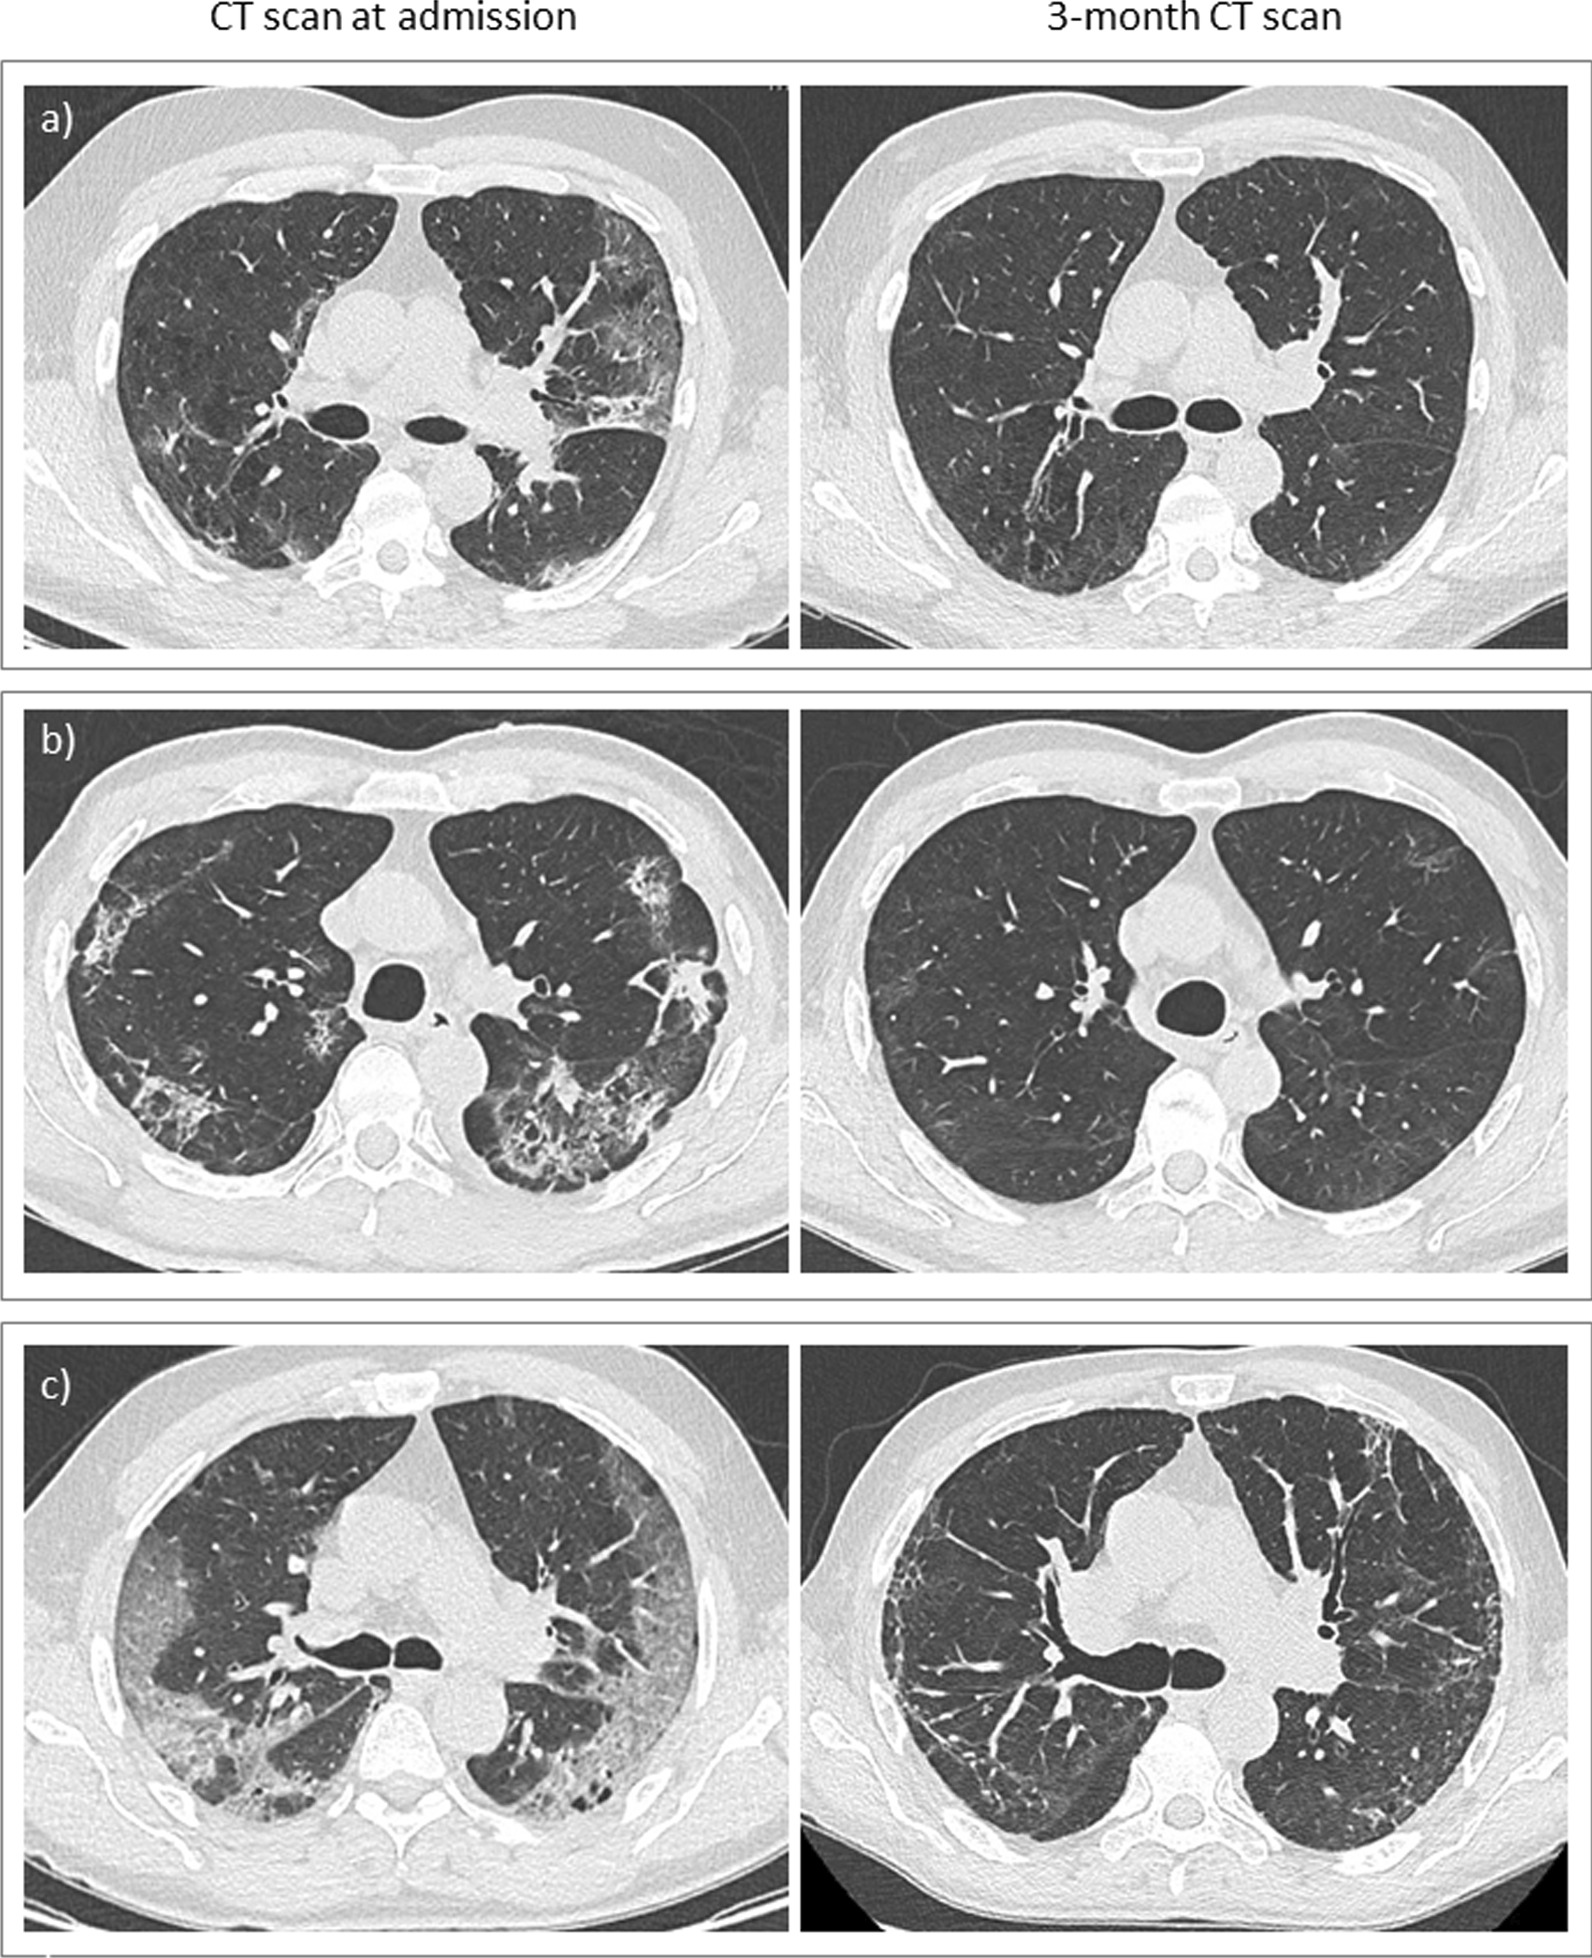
CT-scan features at diagnosis and 3-month assessment in patients with decreased CT-score (a, b) and patients with increased CT-score (c) at 3 months
